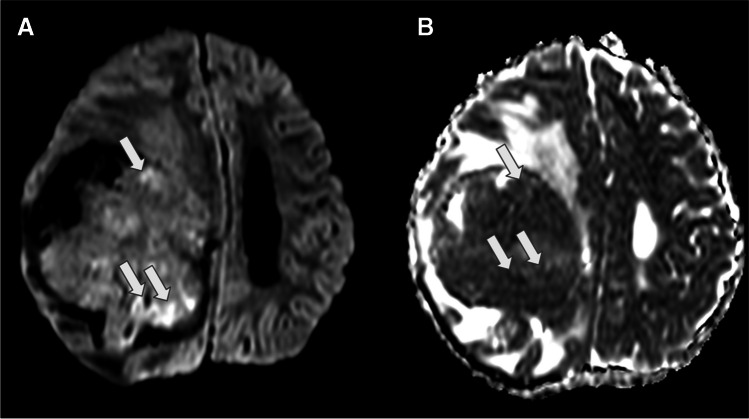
Intraventricular meningioma in the right lateral ventricle in a 9-year-old girl with minimal diffusion restriction. The large mass demonstrates a few areas of increased DWI signal (white arrows) in A, with corresponding low values on the ADC map (white arrows) in B
